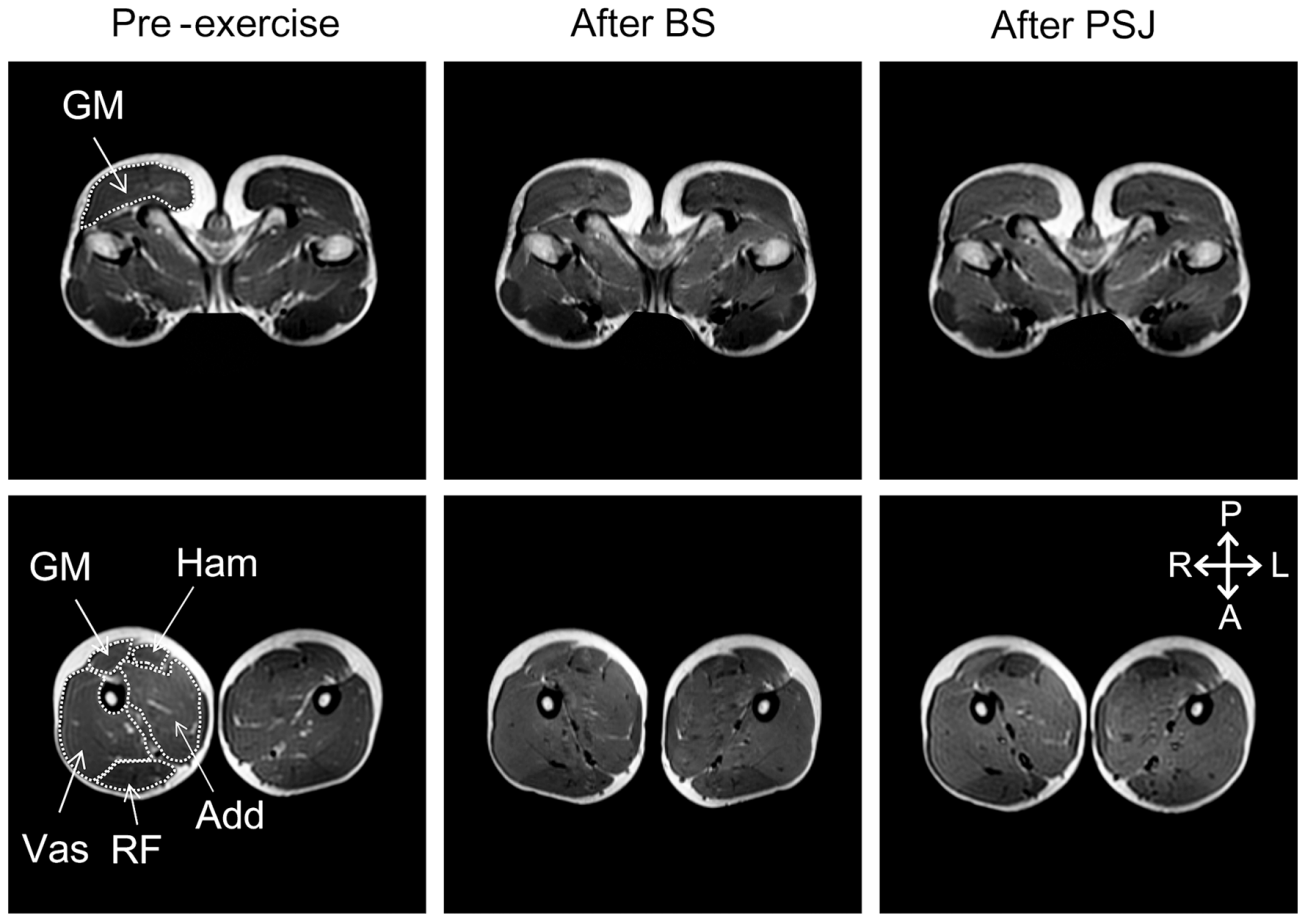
Examples of T2-weighted magnetic resonance images of hip (upper) and thigh (lower) regions at pre-exercise (left), after BS (middle), and after PSJ (right). GM, the gluteus maximus; Vas, the vasti muscles; RF, the rectus femoris muscle; Add, the hip adductors; Ham, the hamstrings. A, anterior; P, posterior; R, right; L, left.
